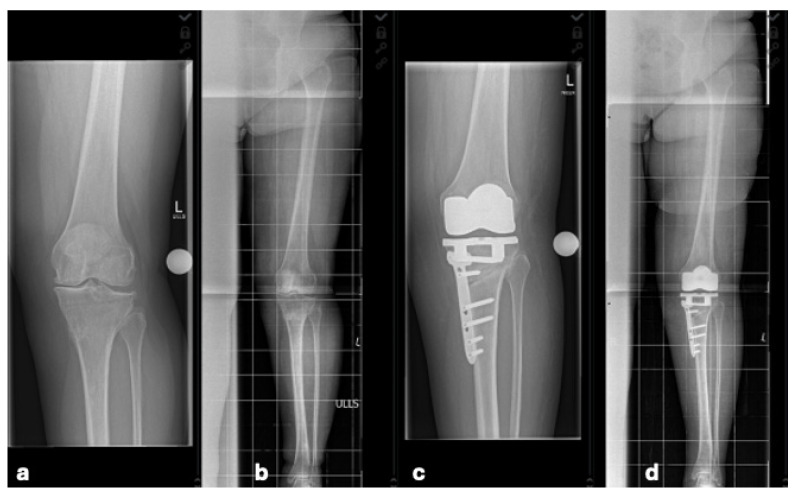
Preoperative and postoperative radiological assessment of a left knee undergoing a single-stage rHTO+TKA. (a) Preoperative anteroposterior knee radiograph and (b) full length standing radiograph showing secondary osteoarthritis and tibial extra-articular valgus deformity. (c) Postoperative anteroposterior knee radiograph and (d) full length standing radiograph demonstrating neutral alignment restoration after medial closed wedge rHTO with a locked plate and TKA. (rHTO: reversed high tibial osteotomy; TKA: total knee arthroplasty).
